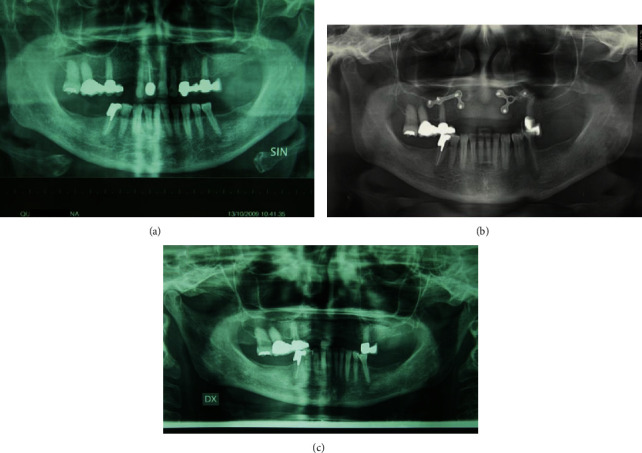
(a) Preoperative panoramic X-ray documenting an osteolytic lesion of the premaxilla. (b) Postoperative panoramic X-ray documenting the correct position of graft and fixation means. (c) Panoramic X-ray after removal of plates and screws (24-month follow-up) revealing signs of resorption and lower radiopacity of the graft material.
